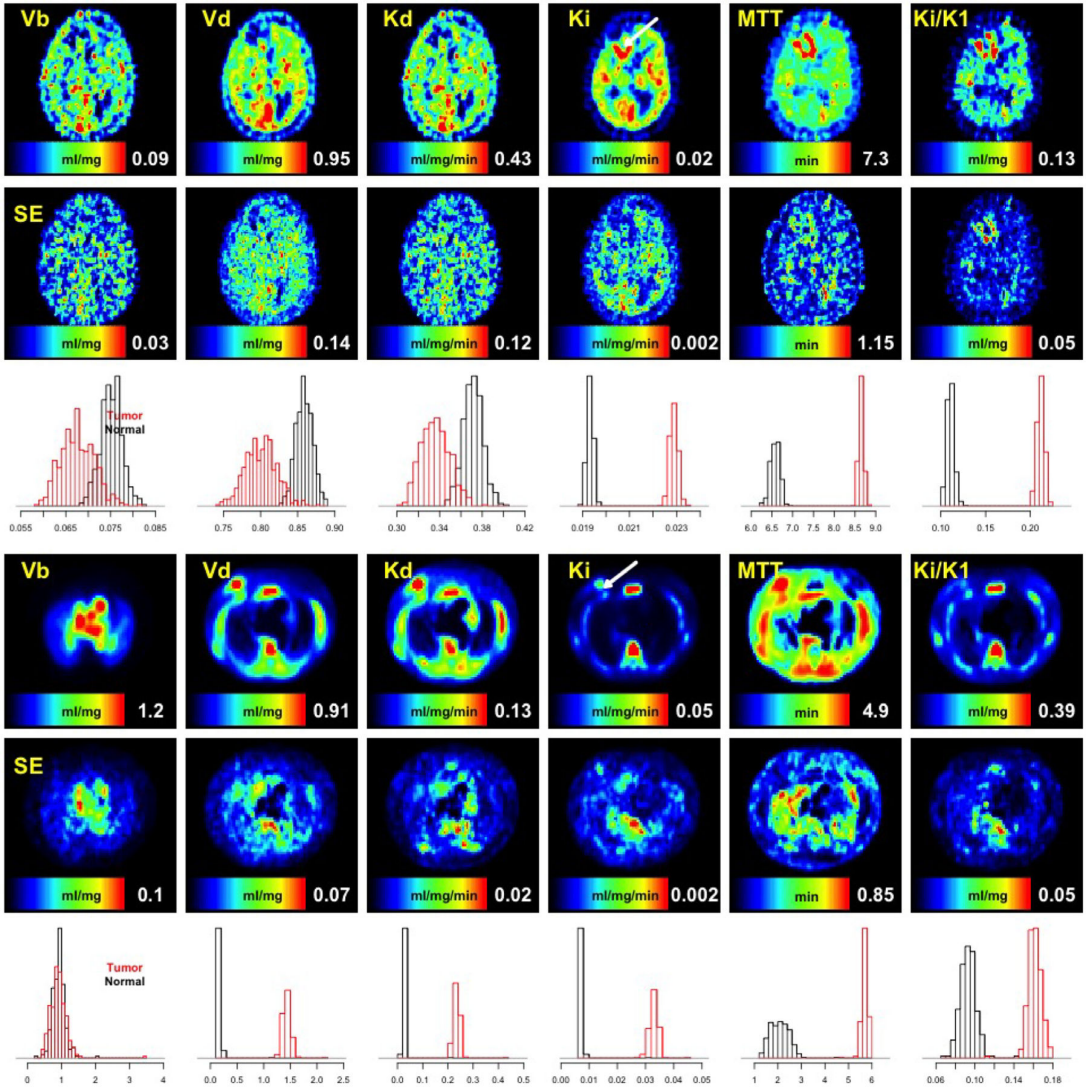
Metabolic Images with image-domain bootstrap assessment of standard errors: Rows 1–3 show the Brain tumor FDG data; 4–6 for the Breast tumor FLT data. The mapped parameters are shown on rows 1&4 – the location of tumor volume of interest (VOI) on the slice is indicated with an arrow. Columns correspond to different metabolic parameters (labeled in yellow) with color bars indicating units - see Section 3.1 for definitions. Computed standard errors (SE) are on rows 2&5. Rows 3&6 show histograms of the bootstrap sampling distributions of the95th percentile of the metabolic parameter in the normal [black] and tumor [red] VOIs.
